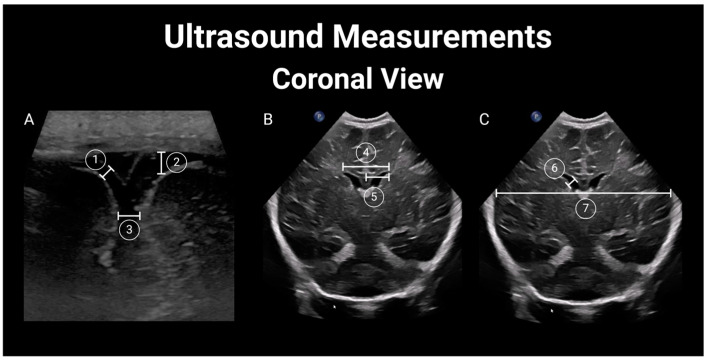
Ultrasound measurements in coronal view. (A) 1. Sino-cortical width (SCW); 2. Cranio-cortical width (CCW); 3. Interhemispheric fissure width (IHW); (B) 4. Lateral ventricular transverse width (VW); 5. Lateral ventricular index (LVI); (C) 6. Lateral ventricle anterior horn width (AHW); 7. Biparietal diameter (BPD).
